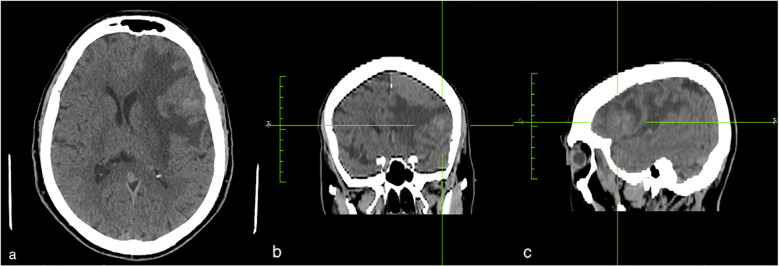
MRI of the brain revealed a meningioma located in the antero-inferior region of the frontal operculum. It measured 21x24x25 mm and was associated with vasogenic edema a transfalcine herniation. The figures show the axial (a), coronal (b), and sagittal (c) projection of the lesion.
